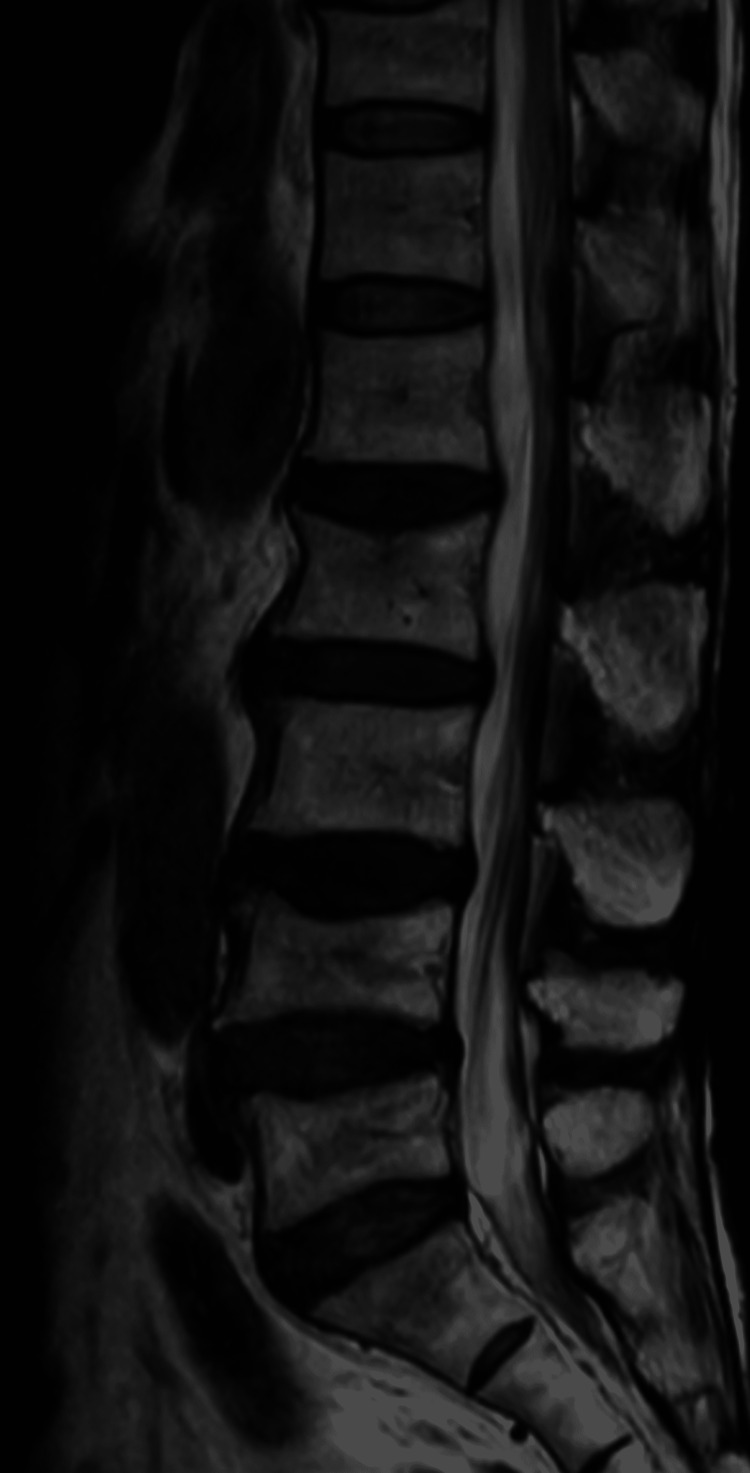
Lumber MRI at three months after onset
